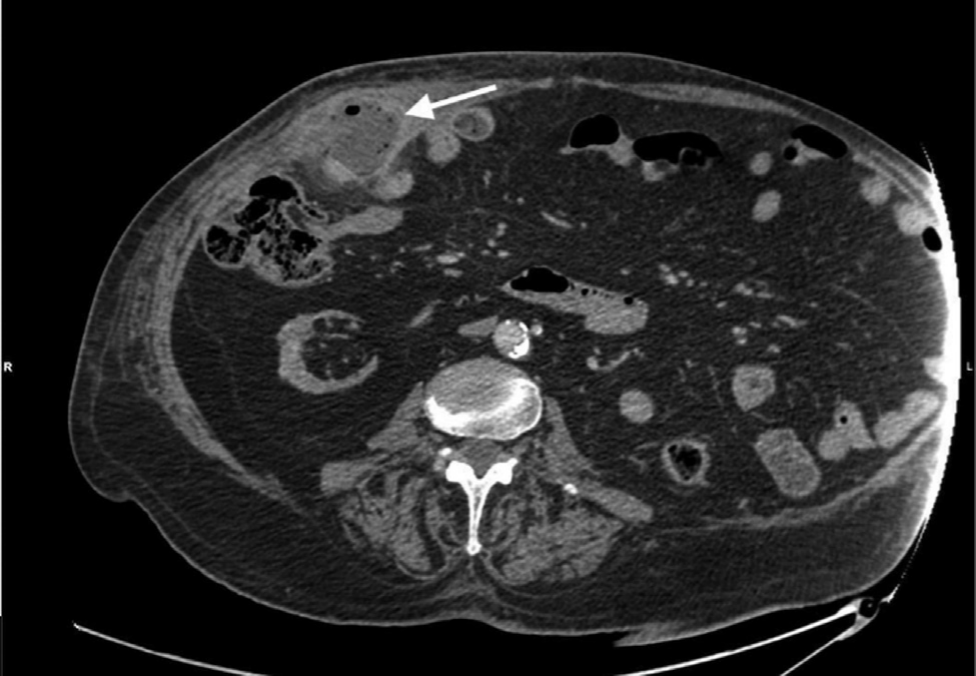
Computed tomography scan showing the peri-appendicular abscess (arrow) of 60 × 40 mm in the Spigelian hernia.
